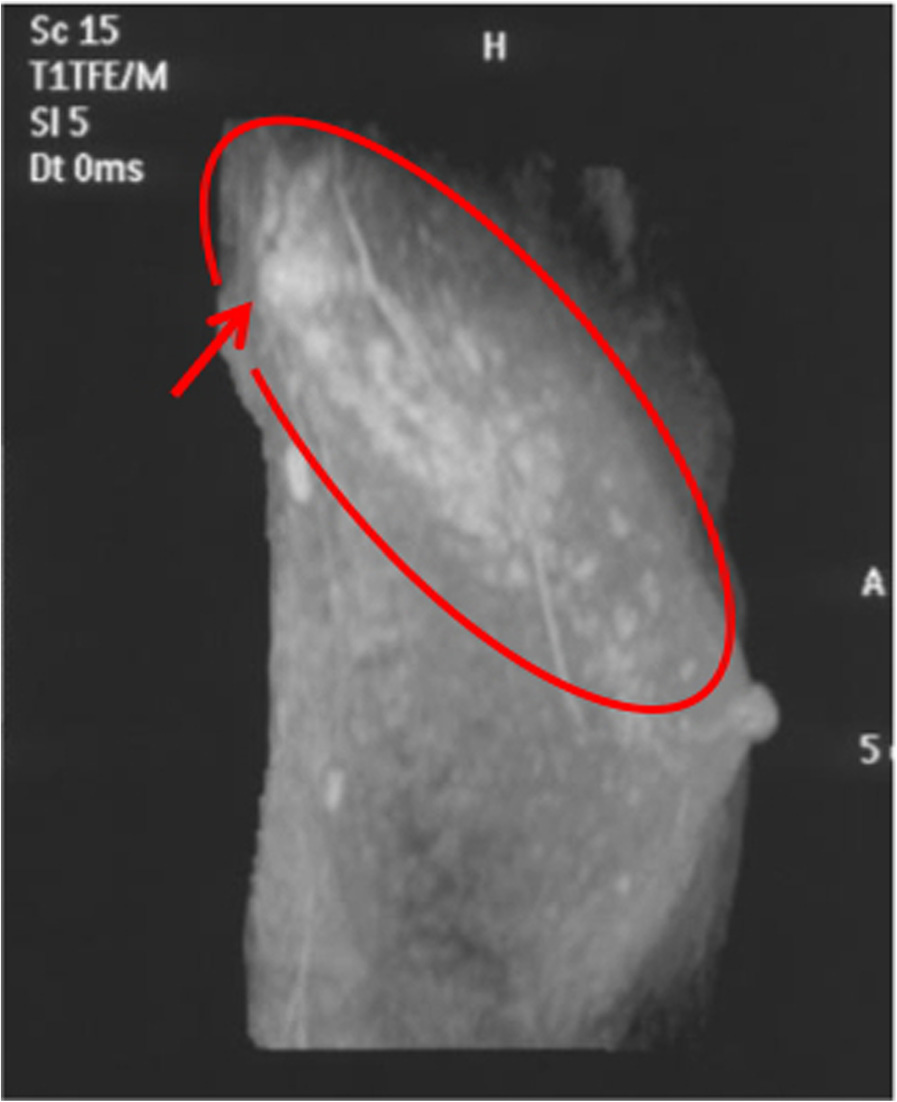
The circle in this breast magnetic resonance image indicates an enhanced area in the upper outer quadrant of the breast, and the arrow indicates the axillary tumor.
